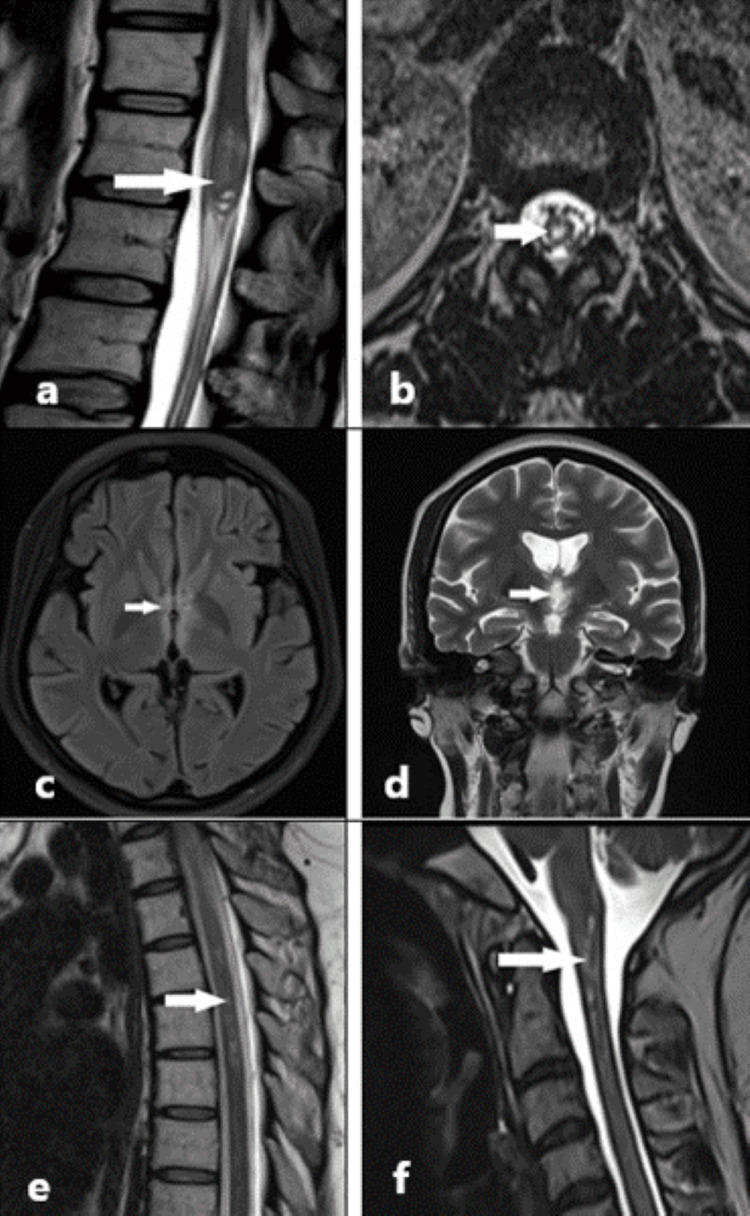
Magnetic resonance imaging of the spine and brain 1a: T2-weighted sagittal image of the spine at the level of the conus medullaris showing hyperintense areas in the conus medullaris (white arrow). 1b: T2-weighted axial image at the level of the conus medullaris showing hyperintensity in the conus medullaris (white arrow). 1c: Fluid-attenuated inversion recovery (FLAIR) sequence; axial image of the brain showing periventricular hyperintensity around the third ventricle (white arrow). 1d: T2-weighted coronal image of the brain showing periventricular hyperintensity around the third ventricle (white arrow). 1e: T2-weighted sagittal image of the spine showing long-segment hyperintensities in the dorsal spinal cord extending from the D2 to D6 vertebral levels (white arrow). 1f: T2-weighted sagittal image of the spine showing short-segment hyperintensities in the cervicomedullary junction and upper cervical spinal cord (white arrow).
